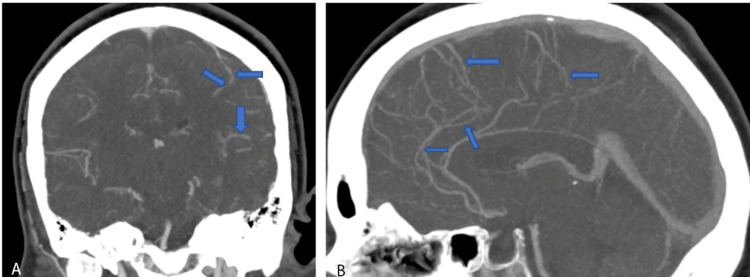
CTA of the head and neck in Case 2 with contrast demonstrating multifocal stenosis of intracranial arteries. CTA: computed tomography angiography
